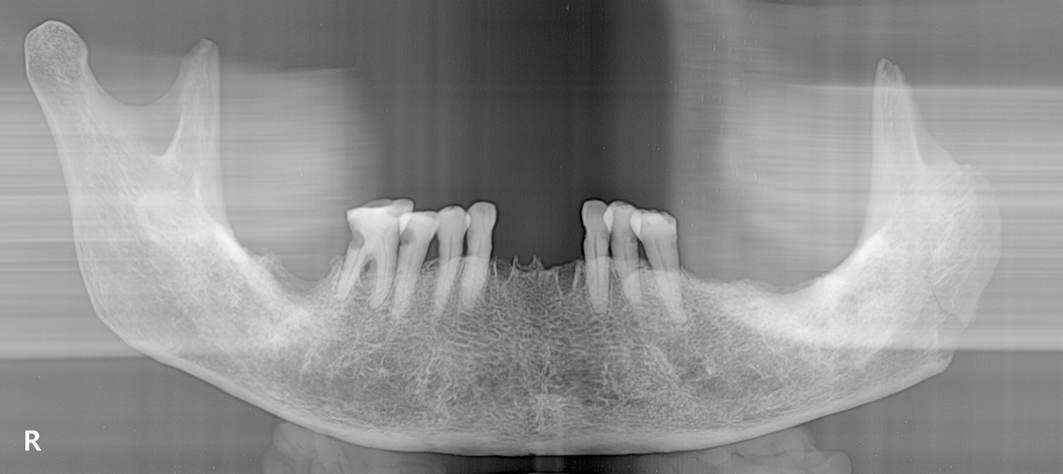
Panoramic radiographs of mandible.
